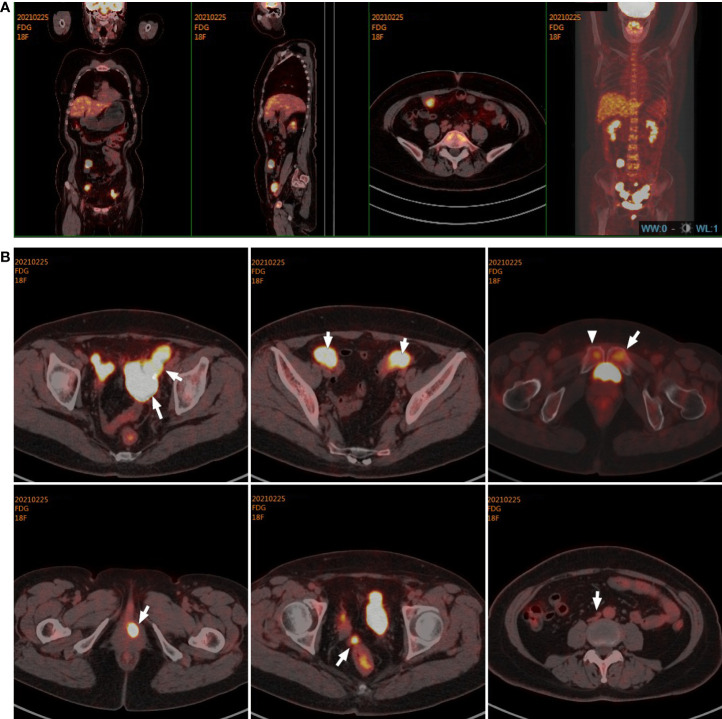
Target lesions of the patient with uterine IMT by PET/CT scans. (A, B) PET/CT scans indicated multiple metastases with increased ^18^F-fluoro-2-deoxyglucose (^18^F-FDG) uptake in the vaginal stump, left-side vulva, abdominal pelvic cavity, mesentery, and iliac paravascular and retroperitoneal lymph nodes in the abdomen and pelvic cavity. IMT, inflammatory myofibroblastic tumor.
